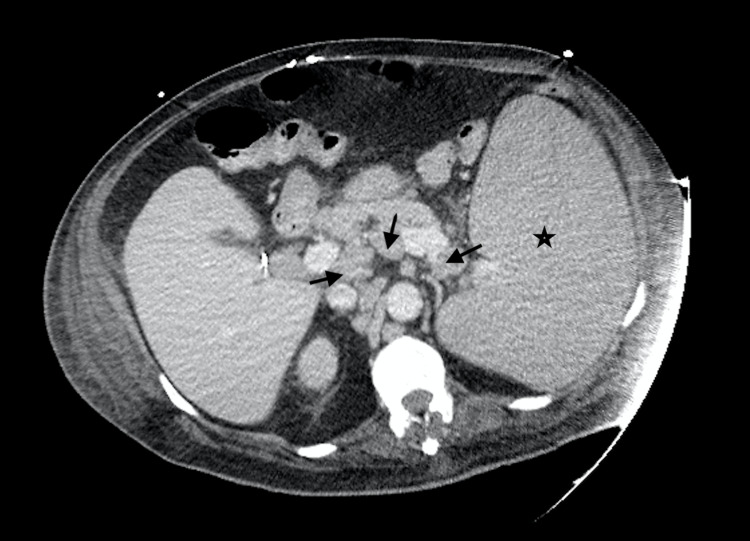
Axial CT abdomen redemonstrating generalized lymphadenopathy (arrows) with massive splenomegaly (star).
